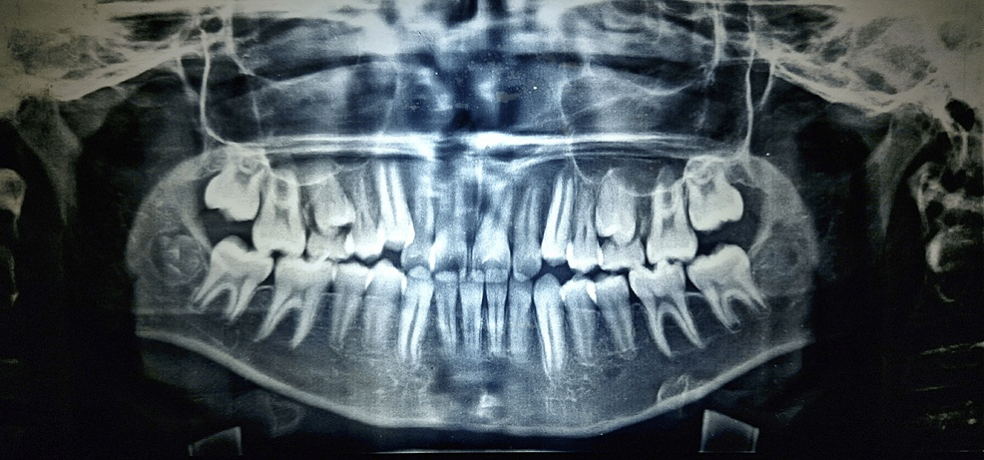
Pretreatment orthopantomogram showing the presence of all teeth except third molars, and the presence of deciduous molars in the first and second quadrants
